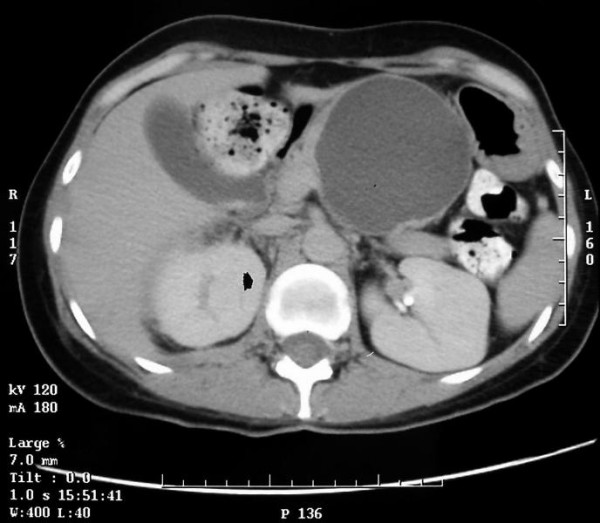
CT scan showing large cystic lesion of the pancreas. Thin walled lesion without segmentation with homogenous fluid content.
